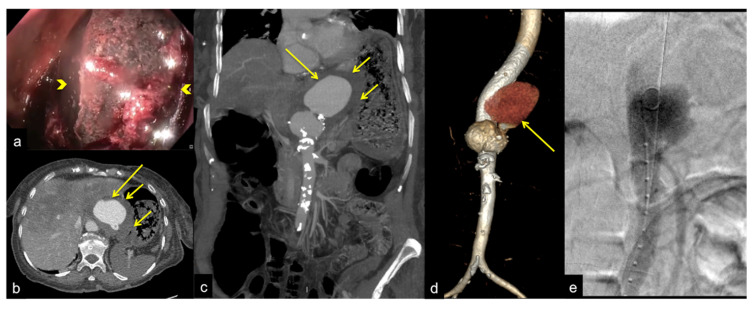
Abdominal pain and anaemia. Gastric endoscopy (a) shows an extrinsic bulging mass in the gastric corpus with a large adherent blood clot ((a) arrowheads). Axial CTA artery phase (b) and coronal MIP artery phase reconstruction (c) show a contained rupture of a thoraco-abdominal aortic aneurysm ((b,c) long arrows) with periaortic haematoma compressing the posterior gastric wall with loss of interface fat plane ((b,c) short arrows). The VR-3D artery phase image reconstruction of the aortic aneurism (d, arrow) better defines the extension of the aneurysm sac ((d) long arrow) for endovascular operative planning. Angiography endovascular aneurysm repair procedure (e).
